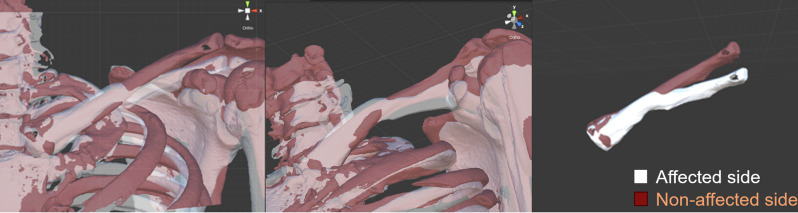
Mirroring and matching of the clavicle. We superimposed the point clouds of the three-dimensional computed tomography image of the left clavicle (white) on those of the mirrored right clavicle image using an iterative closest point algorithm (red) with respect to the first rib. The distal fragment of the left clavicle is displaced inferiorly and is malunited with the left scapula depressed and retracted. The middle panel of the figure shows that the distance between the left clavicle and the left first rib is shorter than that on the right side (up to 7 mm), indicating osseous compression of the left costoclavicular space
